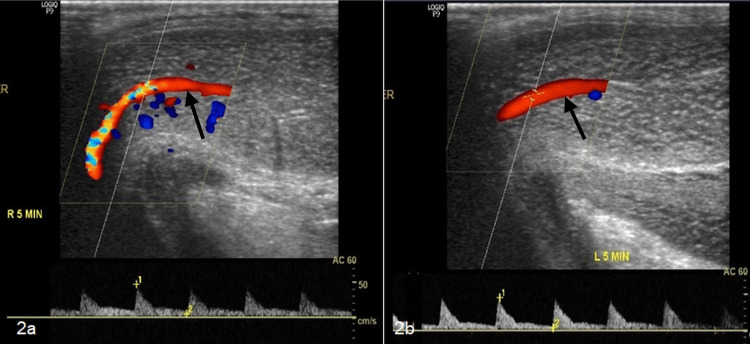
(a and b) Penile Doppler depicting normal blood flow in the dorsal penile vessels (black arrows) on both right and left sides supplying the corpora and glans penis, with no evidence of hematoma.
